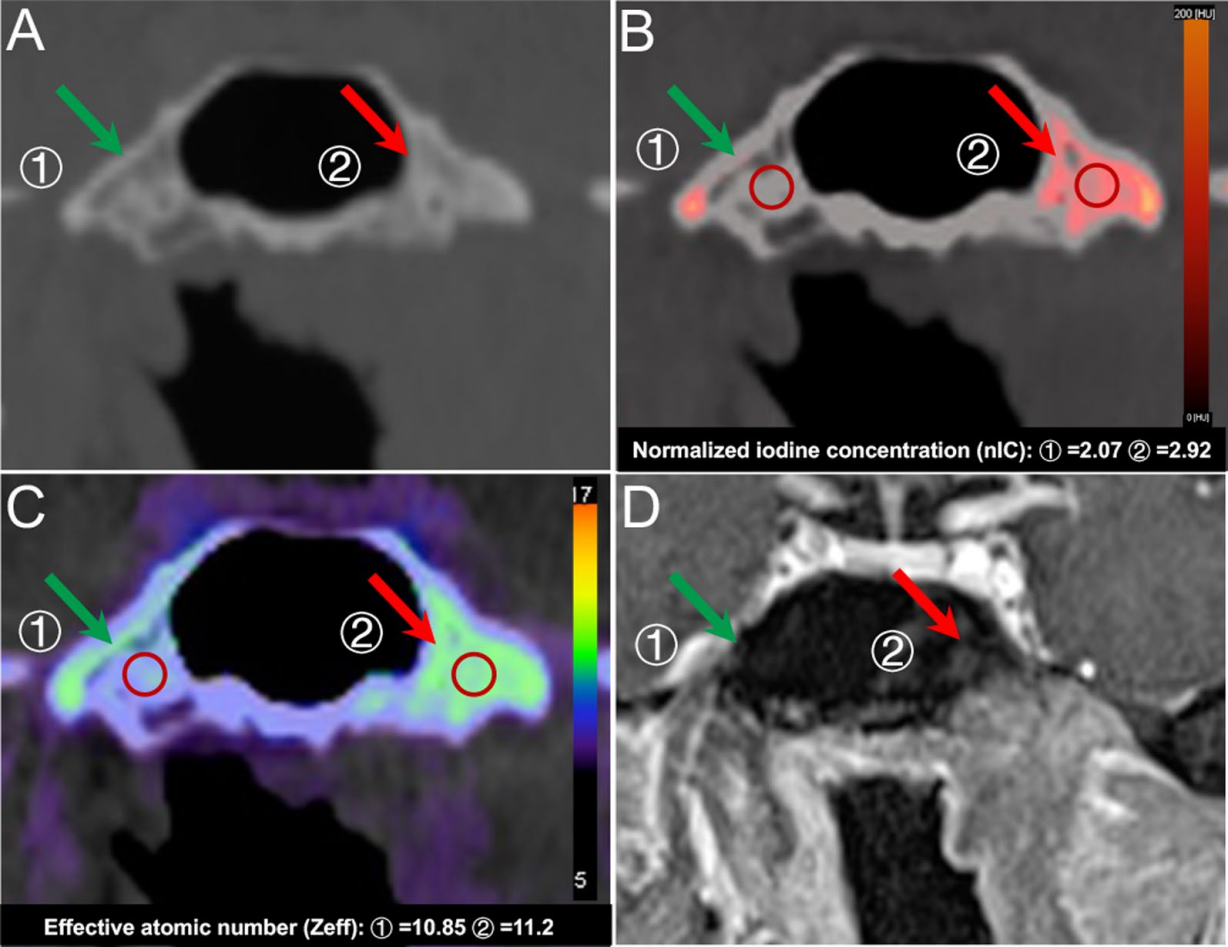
Suspicious false-negative finding for skull base invasions by SECT images in a 54-year-old man with NPC. A The axial SECT images (bone window) show suspicious sclerosis at the left sphenoid body (red arrow). The DECT images (B, IO image; C, Zeff image) directly show marked skull base invasions with higher iodine concentrations than right sphenoid body (green arrow). D The MRI images (fat-suppressed T1-weighted image after gadolinium administration) confirm the same extent of bone invasions as the DECT images with contrast enhancement
